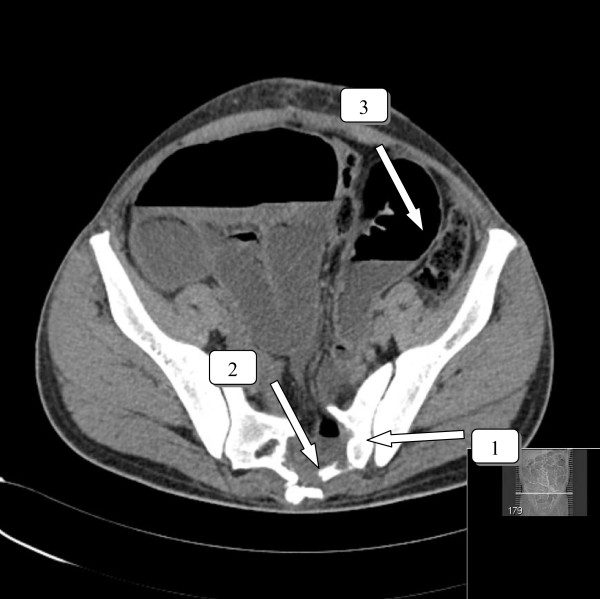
CT scan with axial view. Sacral fracture with entrapment and distention of the ileum and jejunum. 1 sacral fracture. 2 distended entrapped ileum 3. distention of the small intestine.
